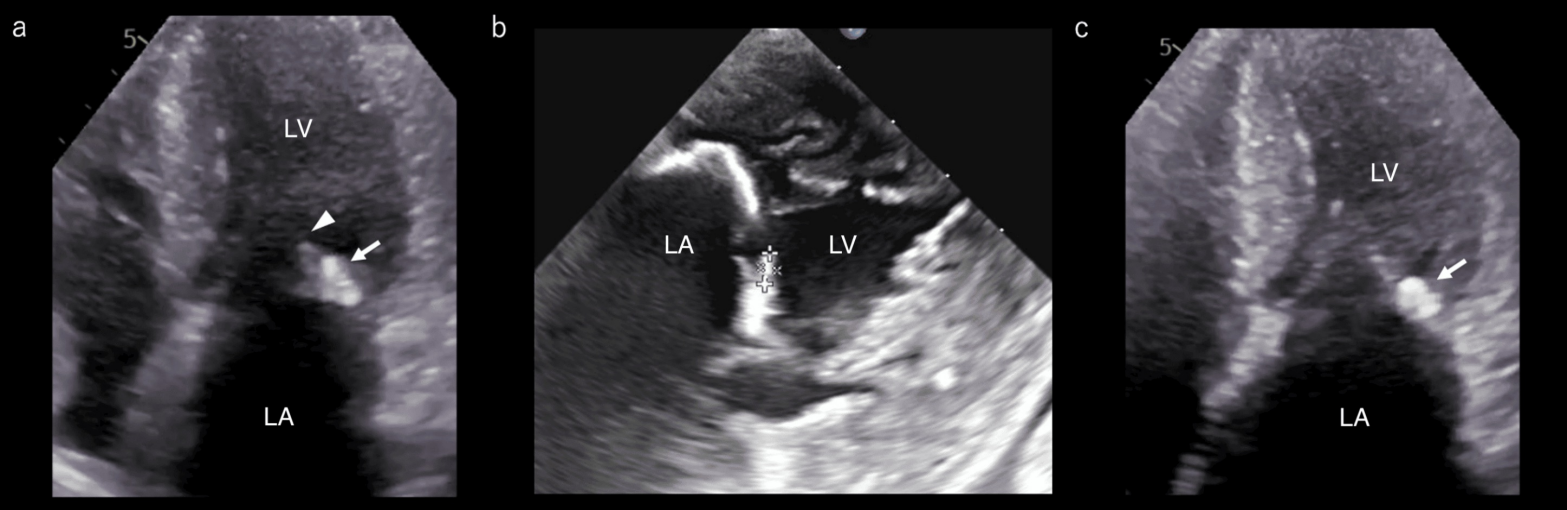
Transthoracic echocardiography (TTE) TTE on hospital day 2 shows mitral annulus calcification (MAC, white arrow), with a mobile mass at the same site (white arrowhead) (a). Transesophageal echocardiogram (TEE) on hospital day 4 confirmed the same findings, revealing a mobile mass measuring approximately 4 × 2 mm (b). Follow-up TTE on post-onset day 53 demonstrated resolution of the mobile mass previously adherent to the MAC (white arrow) (c). LA: left atrium; LV: left ventricle
